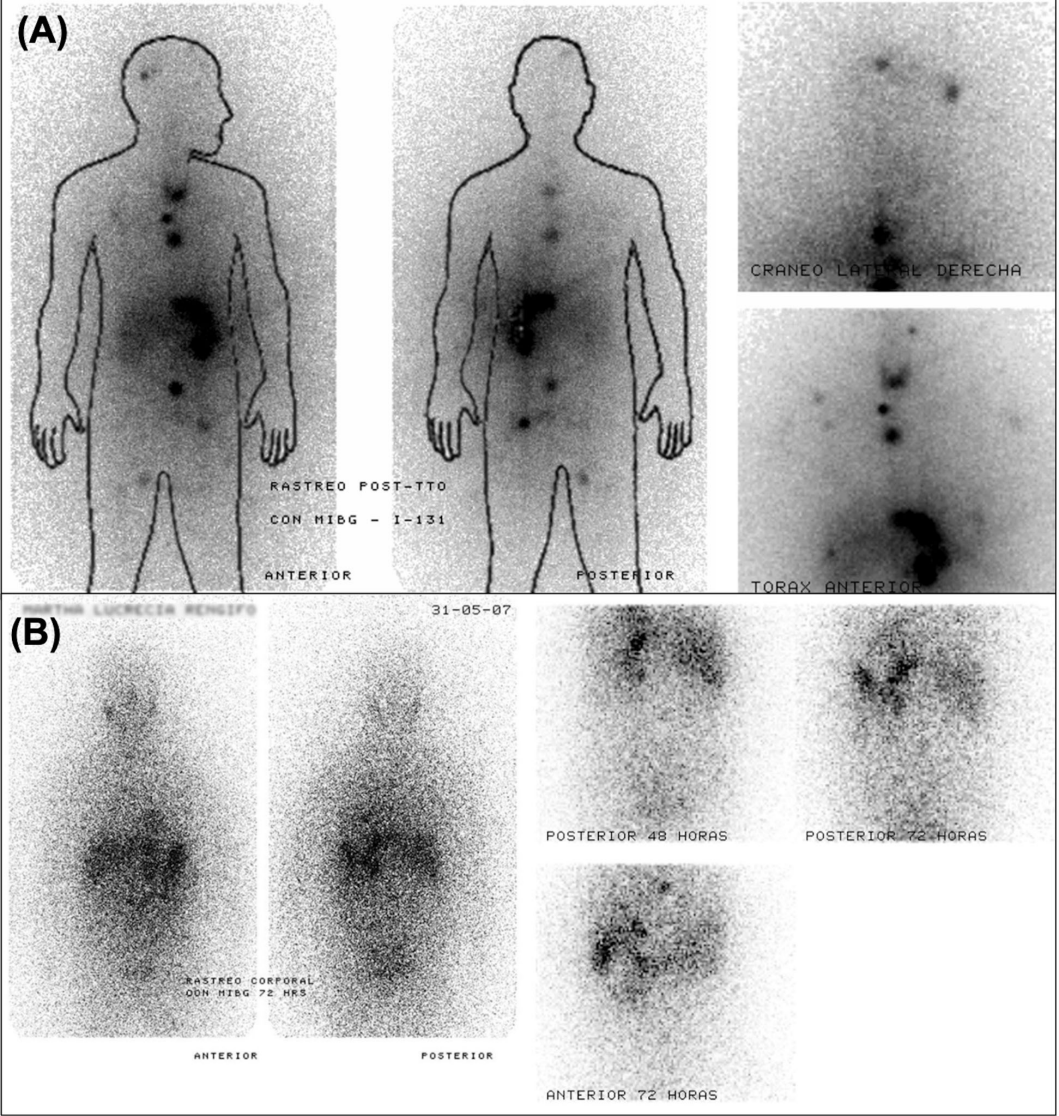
Serial ¹³¹I-MIBG scintigraphy demonstrating partial response to therapy in a patient with metastatic PPGL. (A) Post-therapeutic scan acquired eight days after administration of 300 mCi of ¹³¹I-MIBG shows radiotracer uptake in the skull, mediastinum, retroperitoneum, left internal iliac region, and right inguinal area. (B) Follow-up scan six months later, using 5 mCi of ¹³¹I-MIBG, reveals resolution of most lesions, with persistent uptake only in the mediastinum and liver.
